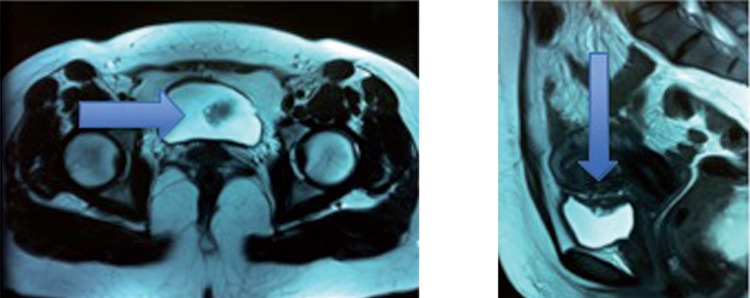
MRI of the pelvis depicting hypo-signal on T2 (lesion is highlighted).
